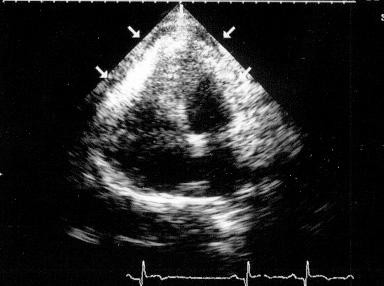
2D-Echo: apical four-chamber view showing a small global echodense pericardial effusion.
